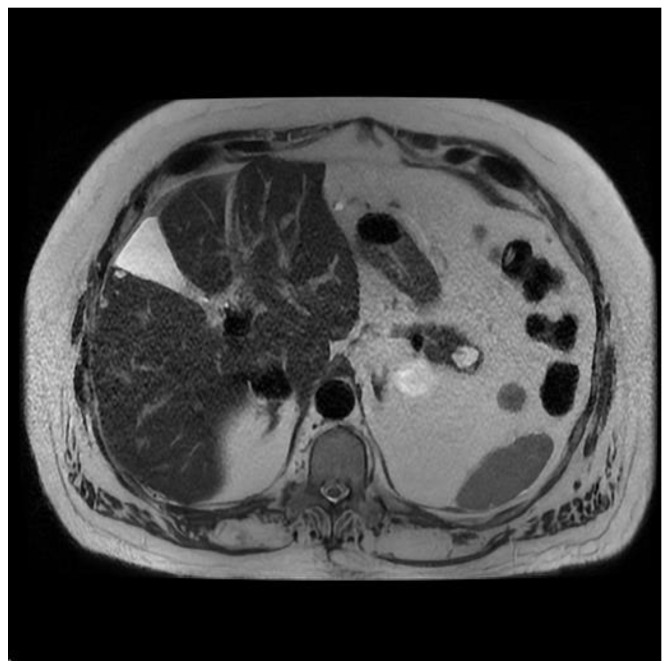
MRI of cNET of the pancreas.
